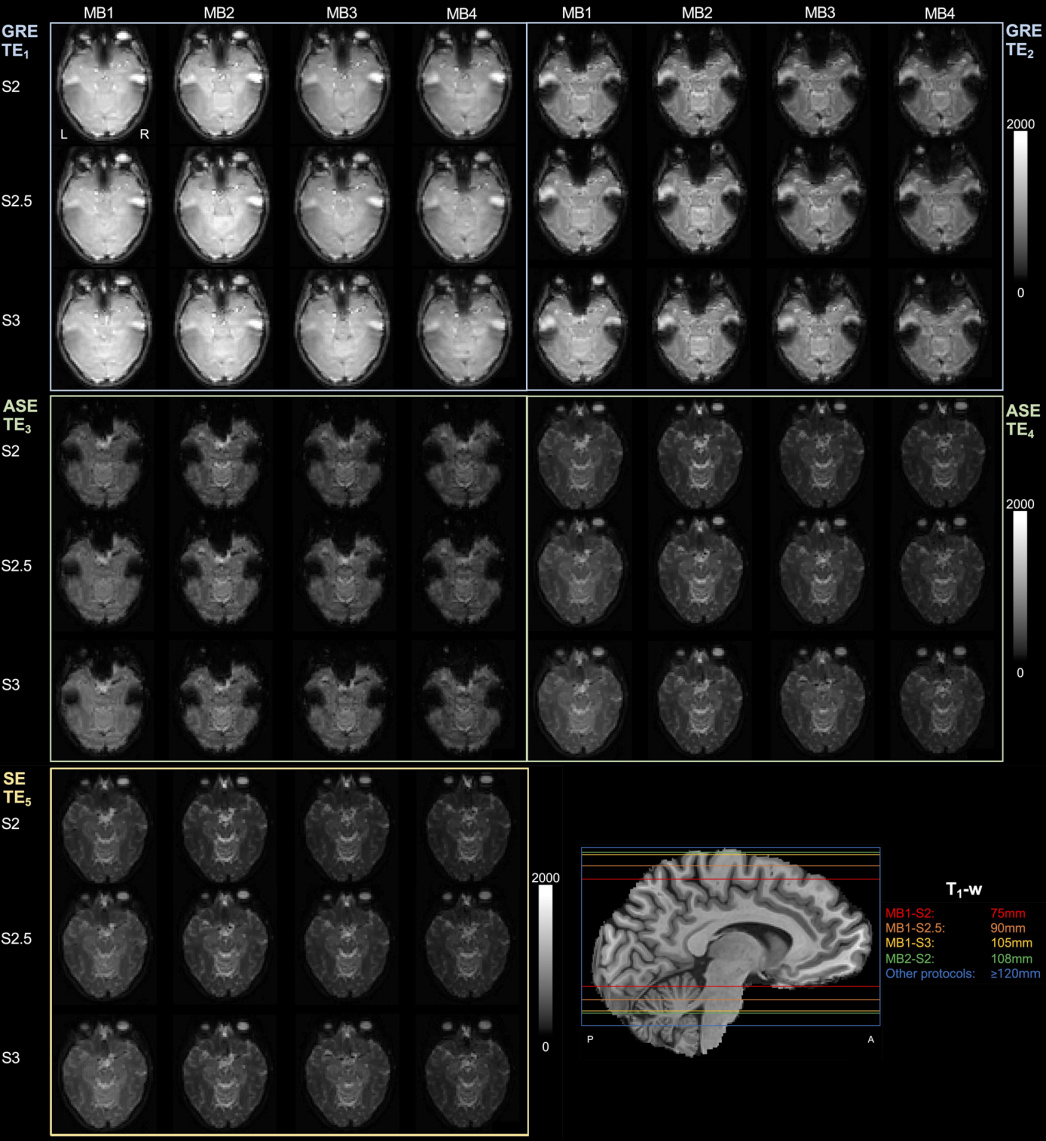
Single-subject acquisition for each TE across acceleration factor combinations. T1-weighted (T1-w) sagittal image is included with each maximum brain coverage achievable per protocol listed in the legend.
